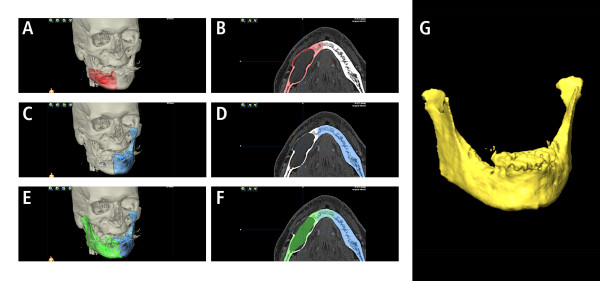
CT scan showing an extended mandibular lesion (keratocystic odontogenic tumor). Swelling of the mandible makes conventionally bending prior to resection difficult (A, B). By Computer assisted pre-operative planning by mirroring of the unaffected left side (template C, D; mirrored template E, F) a stereolithographic model (G) could be printed and a reconstruction plate could be bended prior to surgery.
